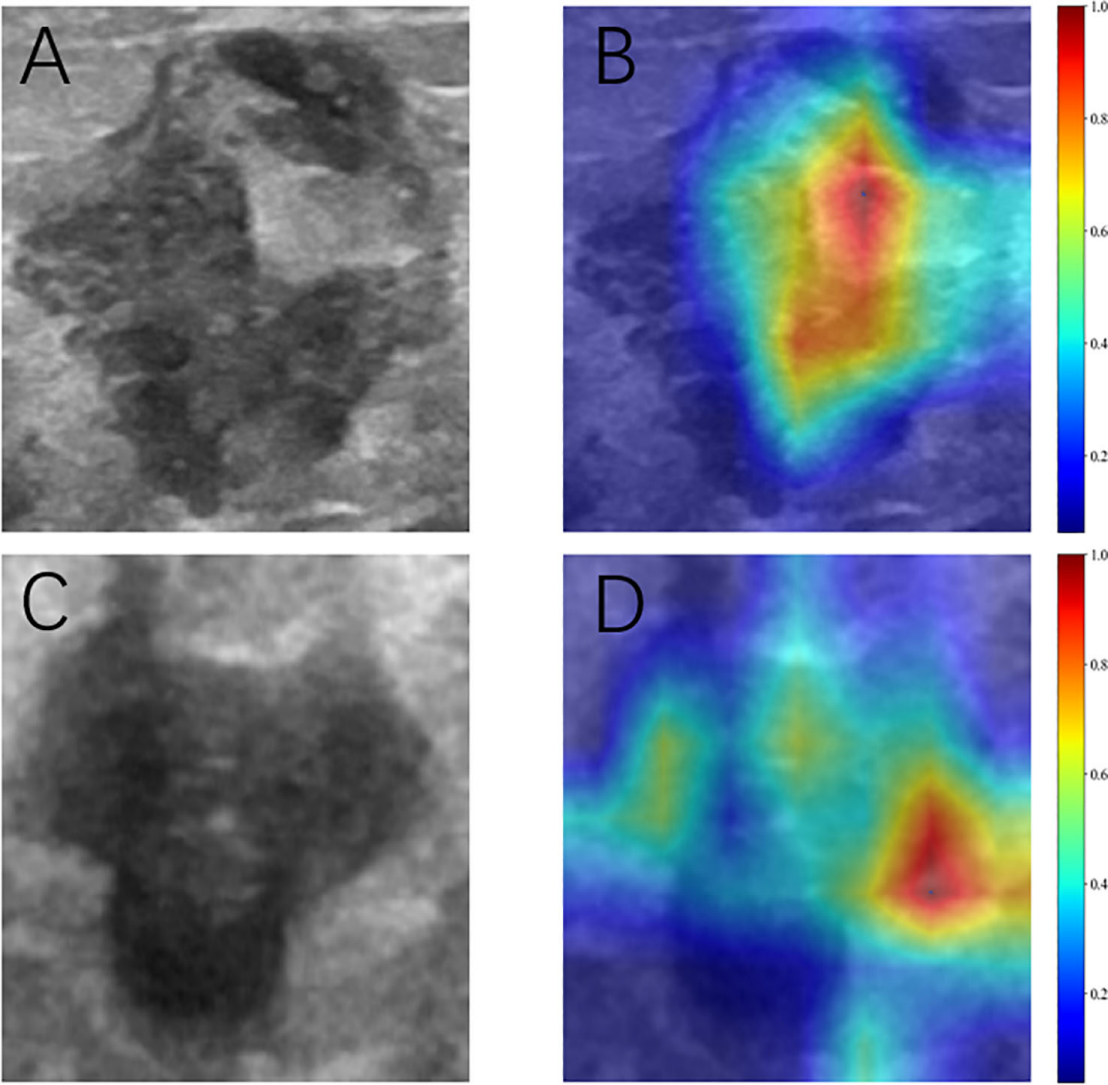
Grad-CAM visualization of two typical samples. (A, C) Ultrasonic images. (B, D) Corresponding heat maps. The red areas indicate higher contributing, and the blue areas indicate lower contributing for predicting SLN metastasis.
